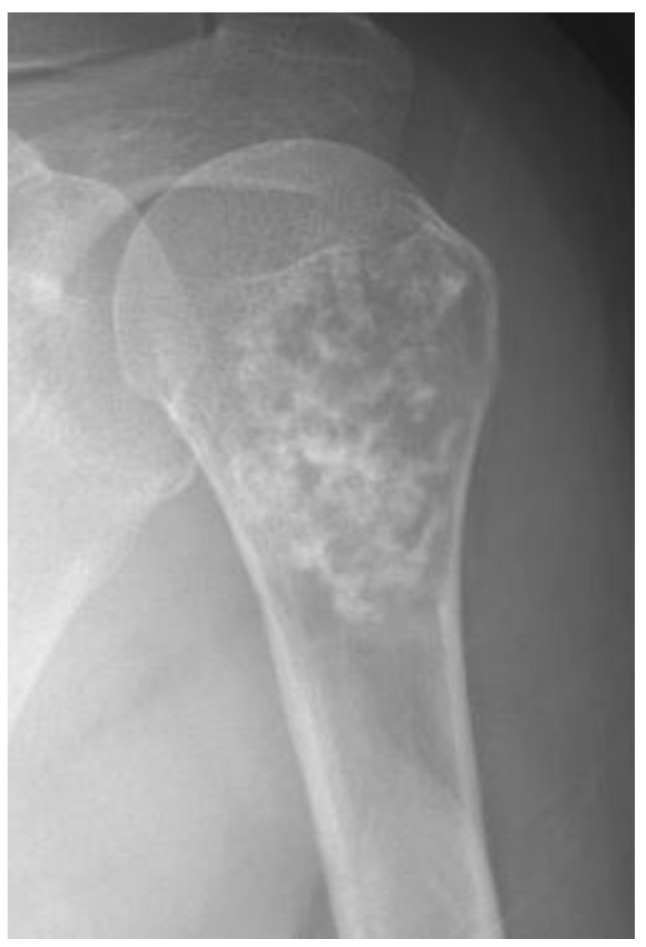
Atypical cartilaginous tumor of the humerus in a 59-year-old woman. Anteroposterior shoulder radiograph shows a mixed lytic and sclerotic lesion in the humerus. The sclerotic component represents typical chondroid ring-and-arc calcification.
